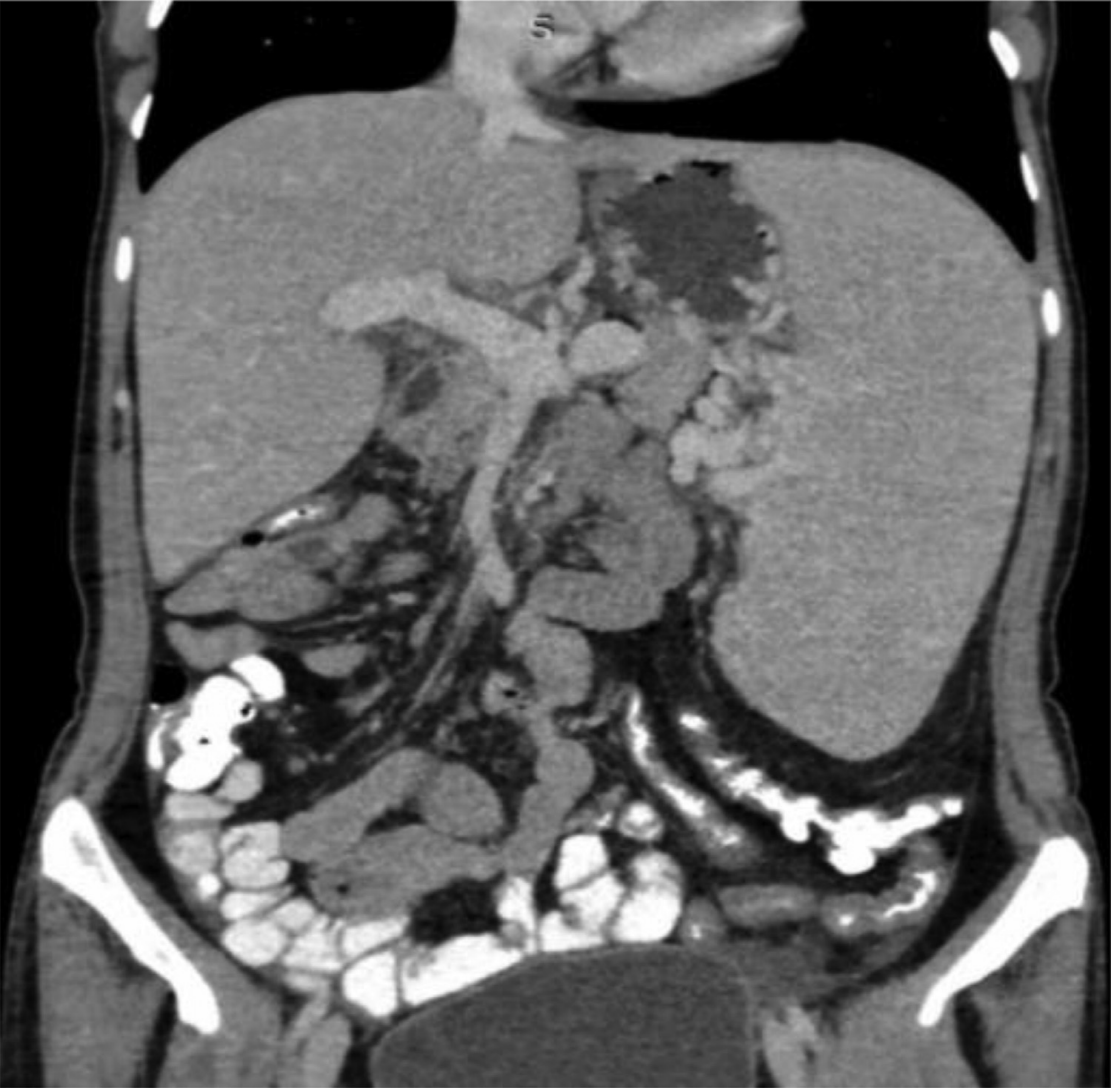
A CT scan with IV contrast revealed an enlarged spleen with peri-gastric and splenic varices, as well as dilated portal and splenic veins. There is no evidence of nodules, irregularities, or any signs of liver cirrhosis.
